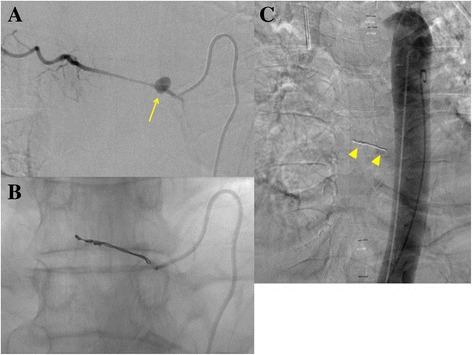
a A pseudoaneurysm of the right seventh intercostal artery (*arrow*) was confirmed by digital subtraction angiography at the same location as on enhanced computed tomography. b After embolization. c Aortography revealed the positional relationship between the microcoils (*arrowhead*) and the descending aorta
